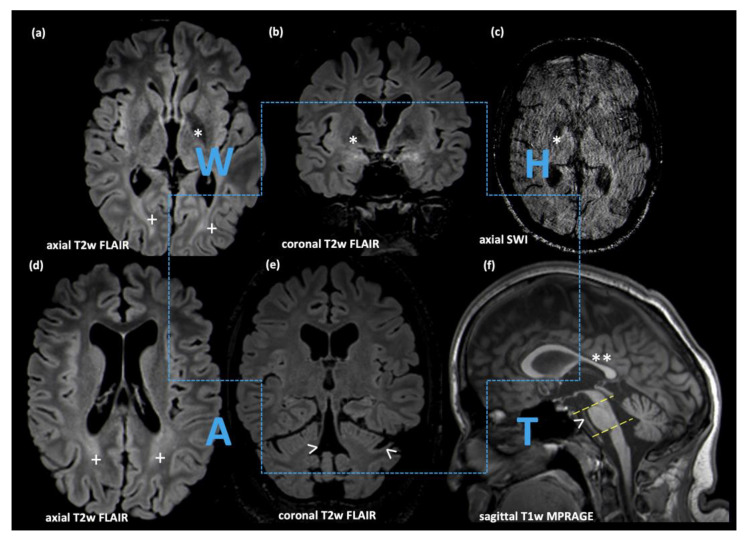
MRI Findings. “WHAT” tetrad: spotty FLAIR-hyperintensities affecting the parietal white matter (“W”; cross symbol in (a,d)), hypointensity of the globus pallidus in T2w FLAIR/SWI (“H”; asterisk in (a–c)), pontocerebellar atrophy with decreased midbrain but not pons midsagittal area (“A”; open arrows in (e,f) (the pontomesencephalic junction is defined by the yellow dotted line between the superior pontine notch and the inferior border of the quadrigeminal plate; the pontomedullary junction is defined by a line parallel to this line at the level of the inferior pontine notch)) and thinning of the corpus callosum, predominantly affecting the body (“T”; double-asterisk in (f)).
